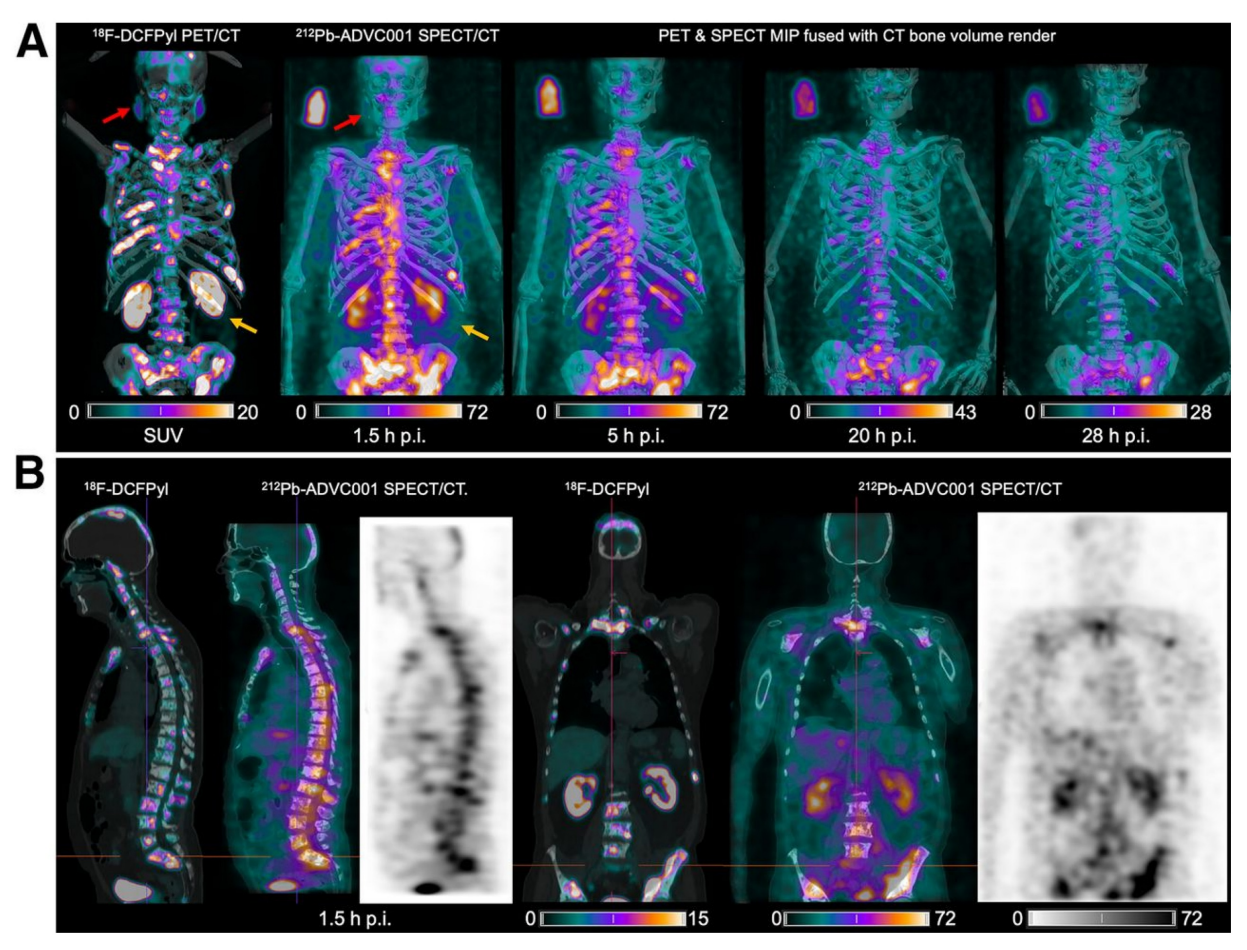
A) PET/CT images taken with [^18^F]DCFPyl concurrently with SPECT/CT images of [^212^Pb]Pb-ADVC001 demonstrating superior dosimetry for salivary glands and kidney clearance for the lead-212 therapeutic. B) Sagittal and coronal images are presented after a 1.5-hour biodistribution time. This research was originally published in *JNM*. Griffiths et al. First-in-human ^212^Pb-PSMA-Targeted α-therapy SPECT/CT imaging in a patient with metastatic castration-resistant prostate cancer. J Nucl Med. 2024;65(4);664. © SNMMI 98.
